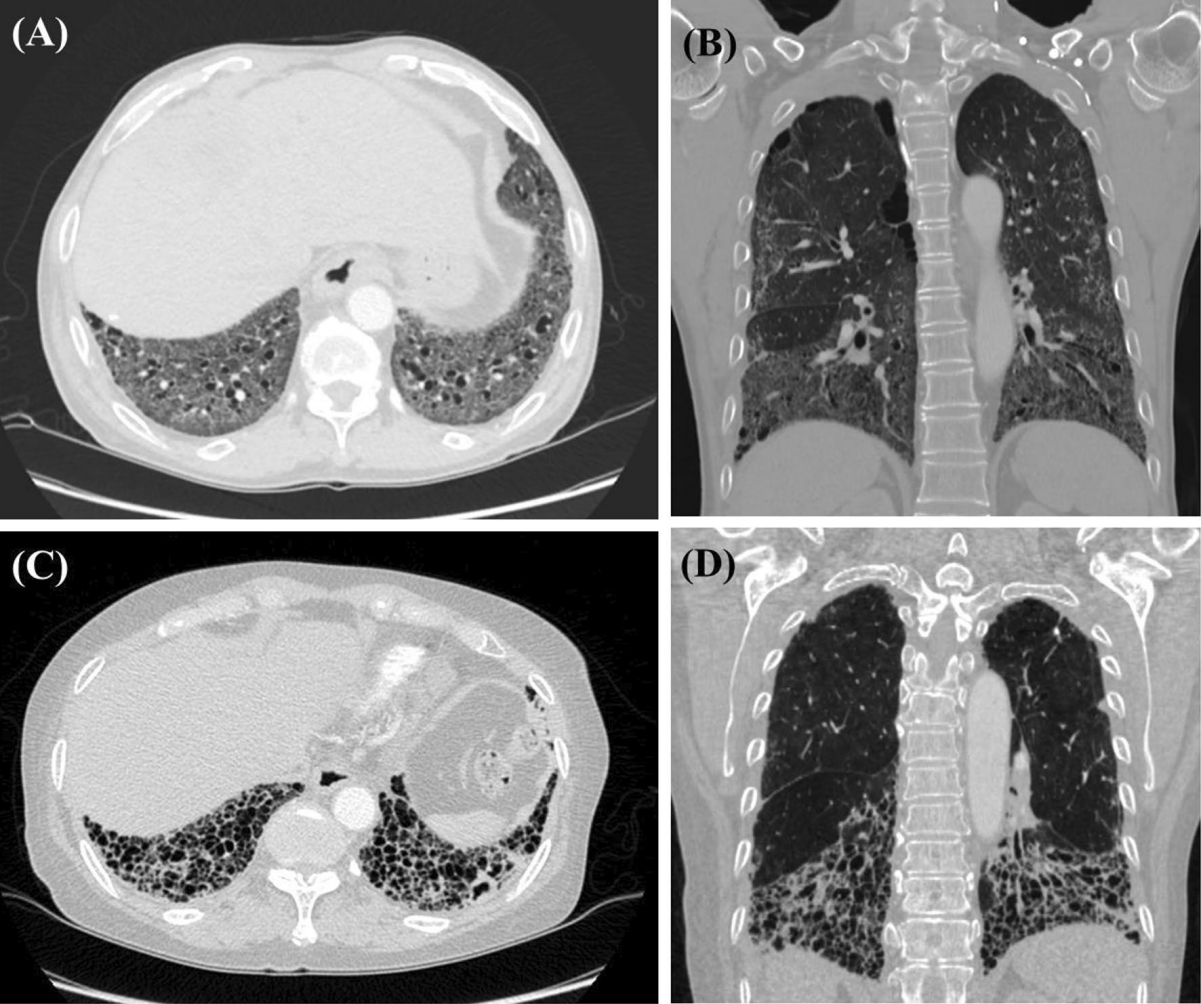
Representative HRCT images of patients with SSc-ILD. Axial (A) and coronal (B) HRCT images of an anti-CCP antibody-negative patient with SSc-ILD. They show extensive ground-glass opacities and traction bronchiectasis with basal predominance. These findings are typical of a non-specific interstitial pneumonia pattern. Axial (C) and coronal (D) HRCT images of an anti-CCP antibody-positive patient with SSc-ILD. They show predominant lower lobe pulmonary fibrosis and traction bronchiectasis with exuberant honeycombing sign. These findings are consistent with the usual interstitial pneumonia pattern. HRCT: high resolution computed tomography; SSc: systemic sclerosis; ILD: interstitial lung disease.
